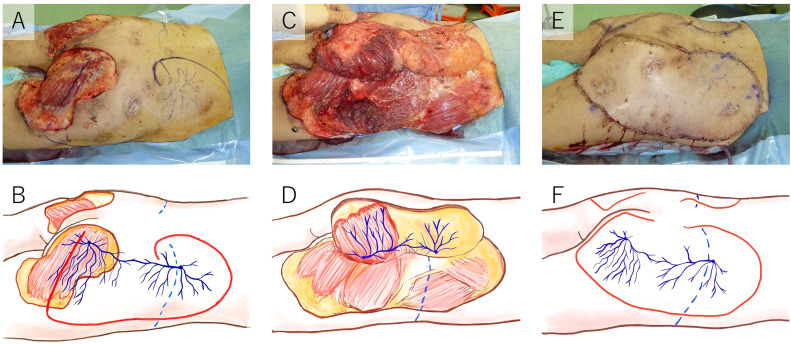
Reconstruction using a combined lumbar artery perforator and split superior gluteal maximus musculocutaneous flap. (A, B) Flap design. The positions of the lumbar artery perforator and the superior gluteal artery perforator were confirmed using Doppler ultrasound prior to surgery. (C, D) Intraoperative findings. The flap was elevated with tissue attached around the lumbar artery perforator for several centimeters. The superficial layer of the gluteus maximus was split to half its thickness and elevated from the distal end to four-fifths of its length, while the proximal one-fifth was left intact. As a result, the superficial branch of the superior gluteal artery perforator was included within the flap. (E, F) Postoperative condition with the defect covered by the flaps.
